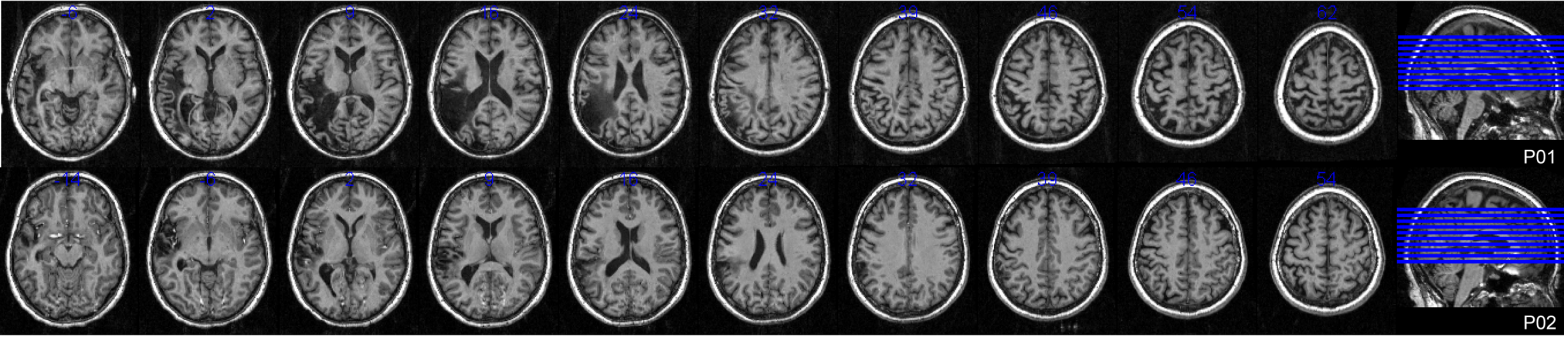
Lesion locations. Axial T_1_ weighted MRI slices at 5 mm intervals for both participants with aphasia.
